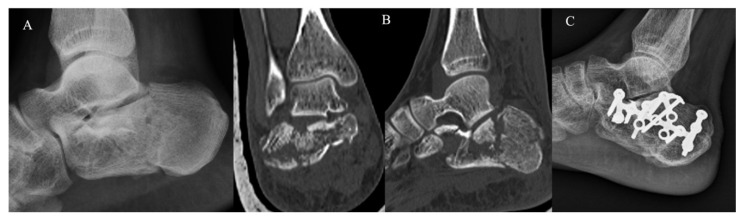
Extended approach and dedicated plate. (A) Pre-operative radiographs; (B) pre-operative CT scan in coronal and sagittal plane showing a Sanders III CF; (C) post-operative radiographs at 4-year follow-up.
